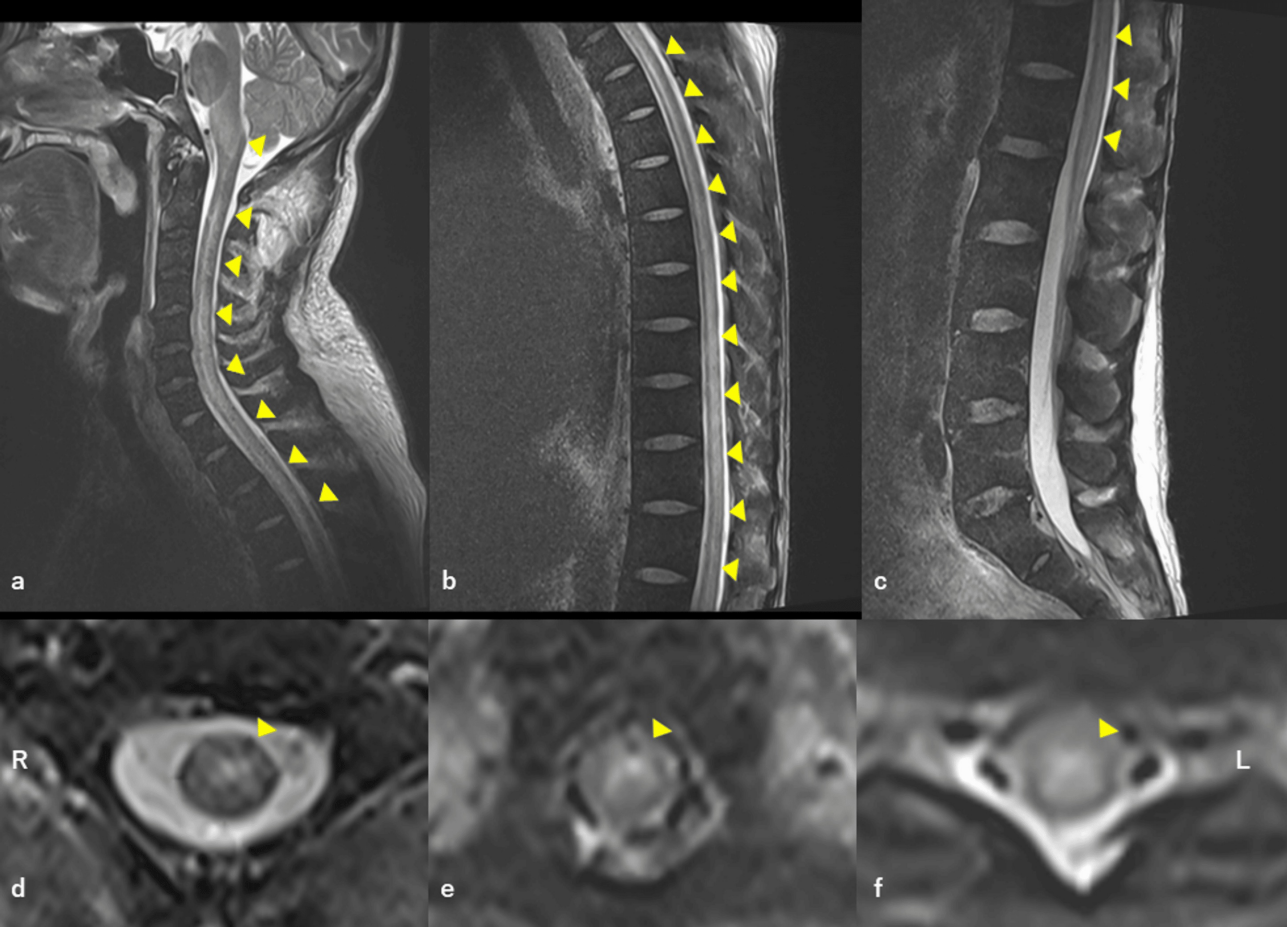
Spinal cord MRI on admission. Sagittal T2-weighted images (a-c). Axial T2-weighted images (d: level of C2 vertebral body, e: level of Th4 vertebral body, f: level of Th10 vertebral body). Long segment (from cervical to lumbar levels) hyperintensity areas in the center of the spinal cord. MRI: magnetic resonance imaging.
